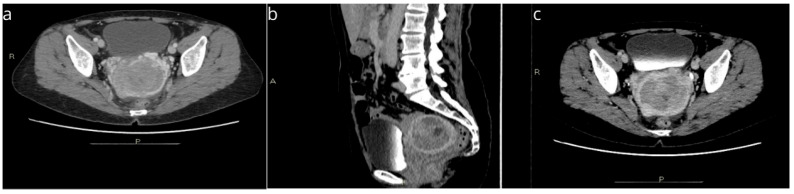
(a) Sagittal CT image demonstrating an enlarged, heterogeneous uterus. A large solid mass with irregular internal attenuation occupies the uterine corpus. No involvement of adjacent pelvic structures is identified. (b) Axial CT image at the level of the uterine body showing the maximum cross-sectional extent of the mass (approximately 76 × 68 mm) with avid peripheral enhancement and central hypodense necrotic areas. The uterine contour is preserved and no evidence of serosal infiltration of adjacent structures is observed. (c) Portal-phase axial CT image confirming absence of peritoneal carcinomatosis, pathological lymphadenopathy, or distant metastatic spread.
